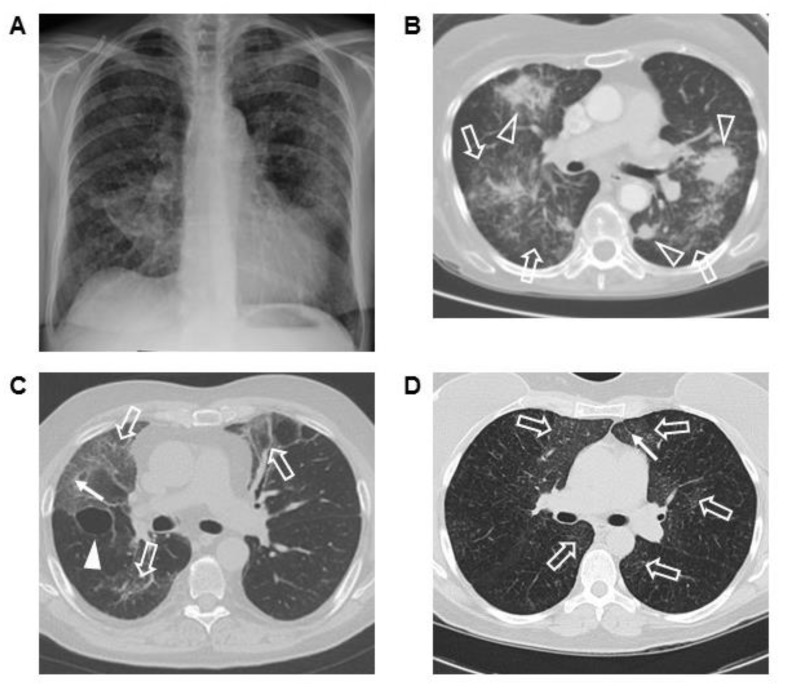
Posteroanterior chest radiograph (A) and representative computer tomography (CT) scan of the chest image (B) from a patient with Erdheim–Chester disease (ECD) and severe interstitial lung disease (ILD). Multiple bilateral consolidated masses (open arrowheads) with ground-glass infiltrates (open arrow) are found (B). Ground-glass infiltrates, reticulations (arrow), and a right lung cyst (solid arrowhead) are shown in another patient with ECD and severe ILD (C). Diffuse ground-glass opacification and reticulations are demonstrated in a third patient (D).
